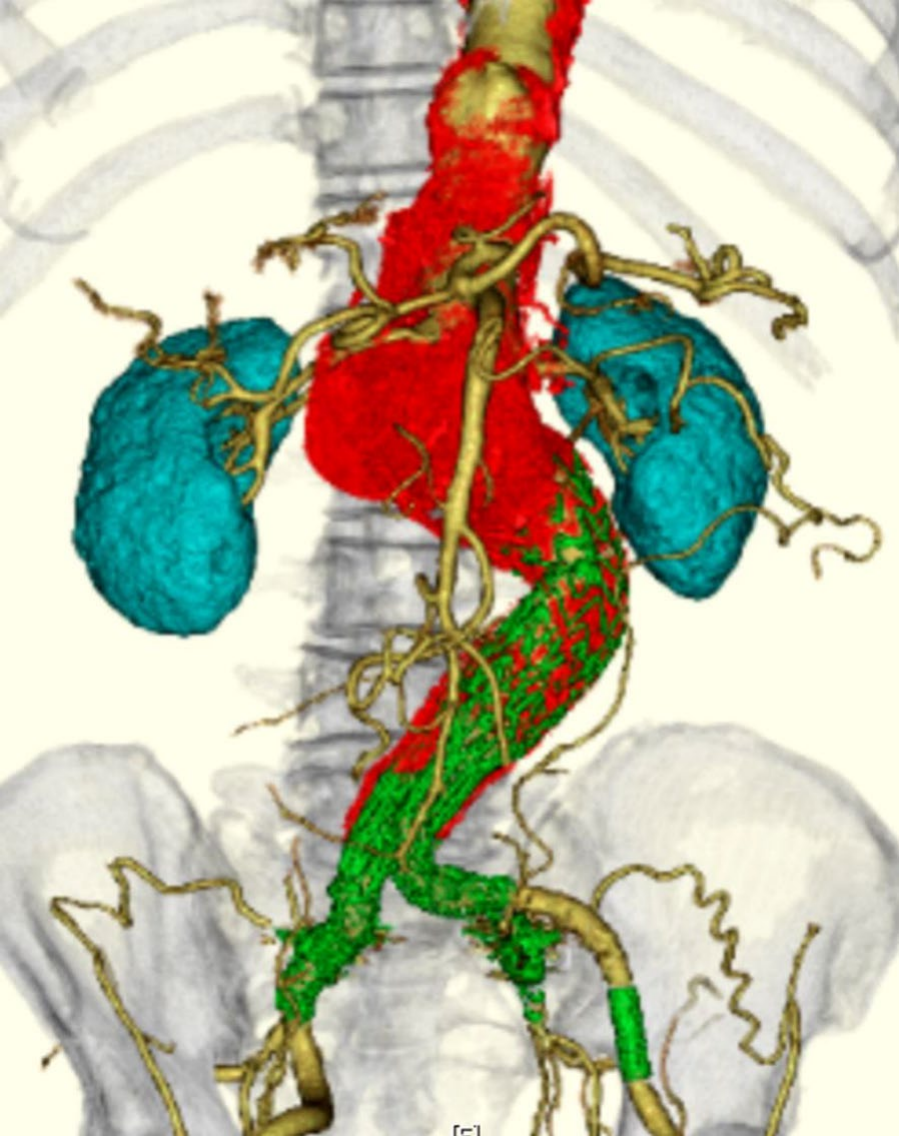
Preoperative 3D computed tomography scan of the first patient
